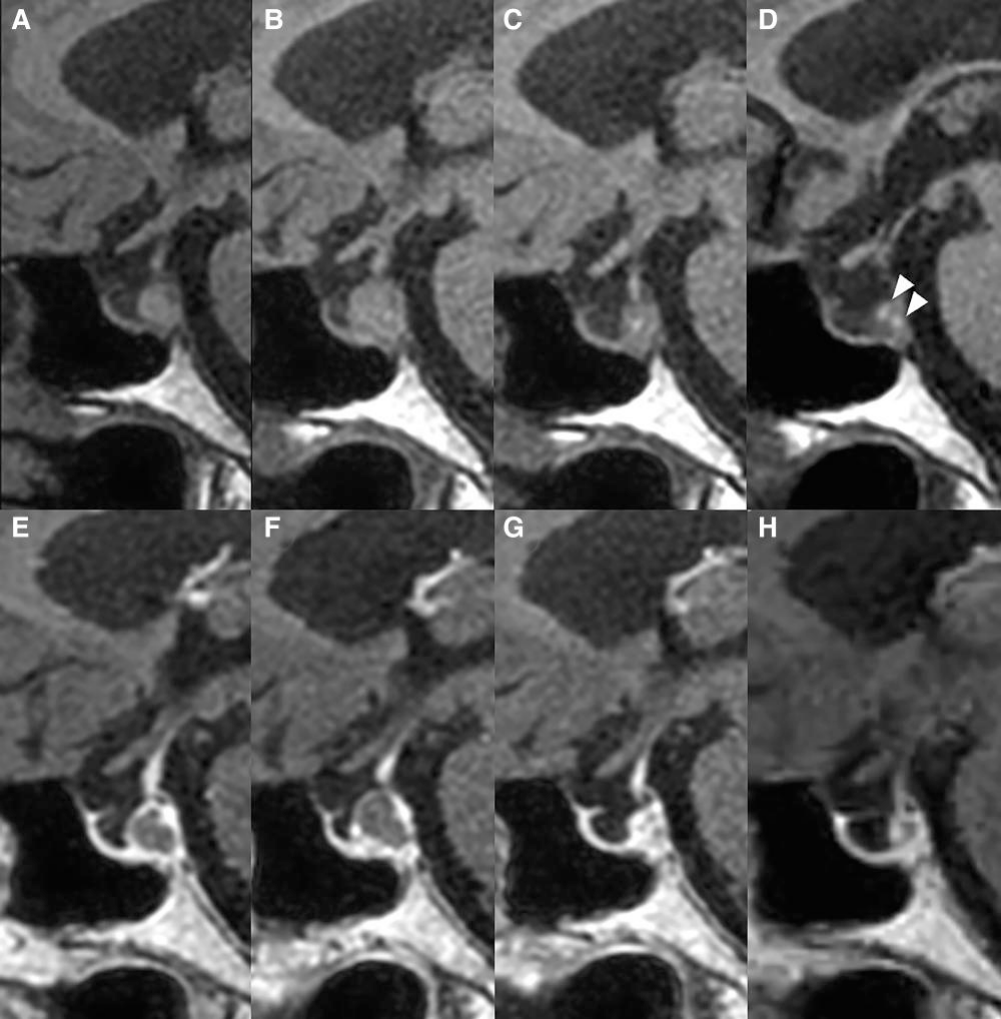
Chronological changes in Rathke cleft cyst (RCC) on T1-weighted magnetic resonance imaging with and without gadolinium enhancement. One week after the initial presentation, spontaneous shrinkage of the RCC was observed along with resolution of headaches (A: noncontrast; E: contrast-enhanced). Three months after the initial presentation, the RCC reenlarged with a recurrence of episodic headaches (B: noncontrast; F: contrast-enhanced). By 6 months, the RCC had reduced in size once again (C: noncontrast; G: contrast-enhanced), and at 9 months, the RCC had further decreased, with a faint reappearance of the posterior bright spot indicated by arrowheads (D: noncontrast; H: contrast-enhanced); however, arginine vasopressin deficiency persisted.
